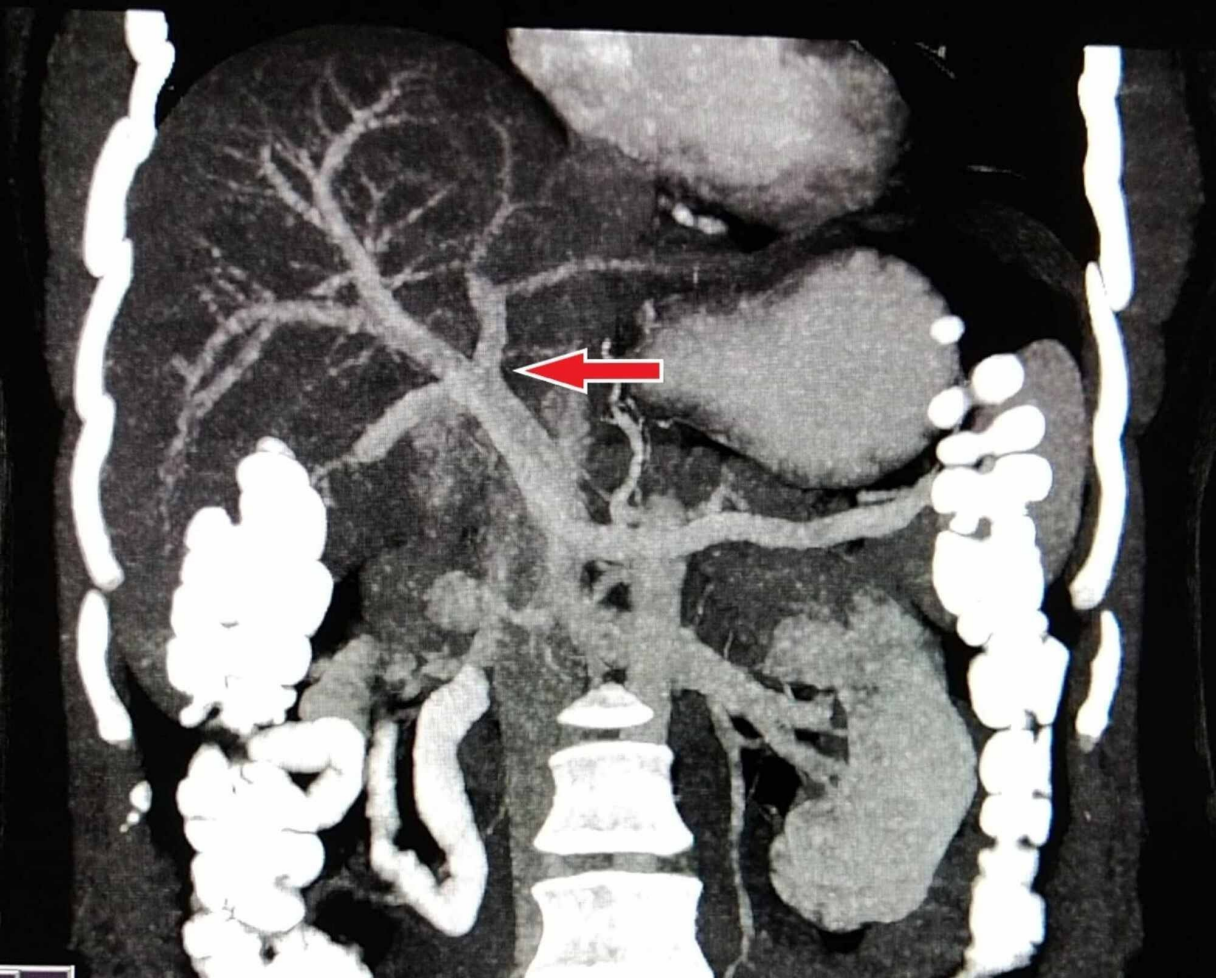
Contrast-enhanced CT MIP reconstructed coronal image showing the trifurcation of the main portal vein (type 2) MIP, maximum intensity projection.
